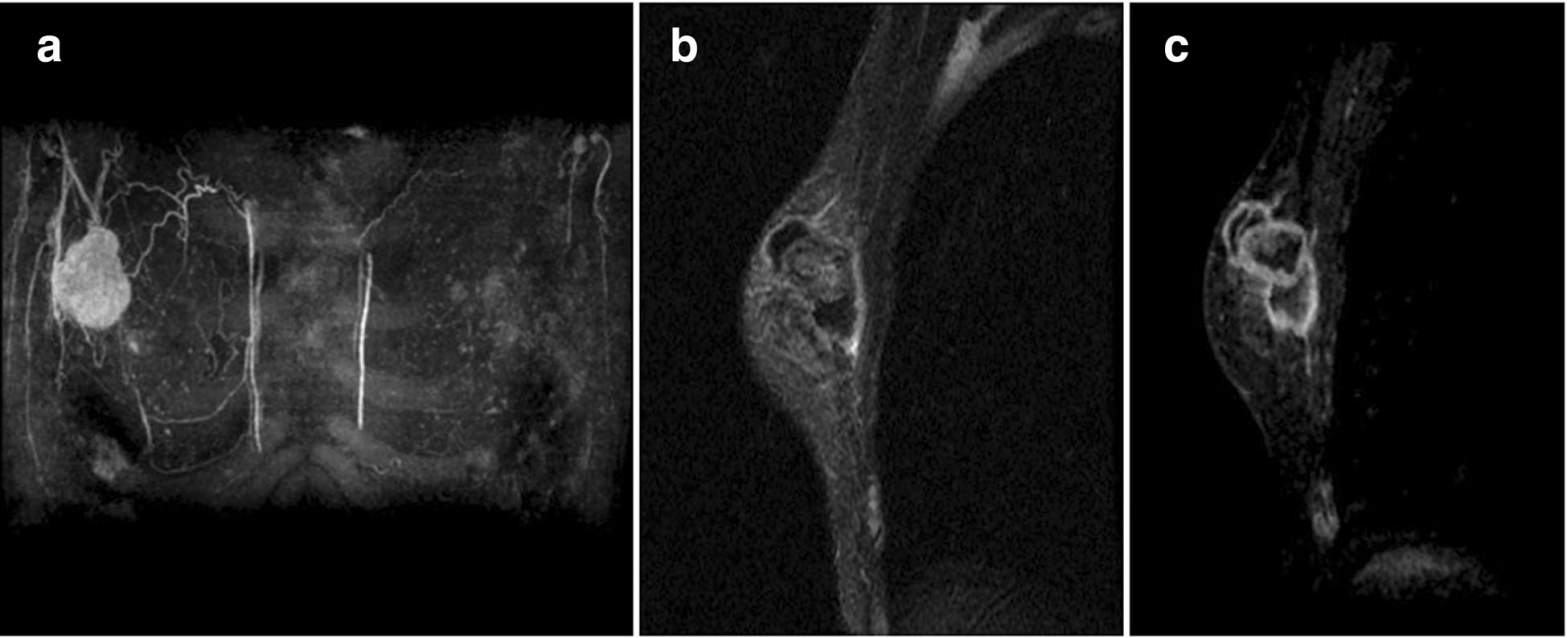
Magnetic resonance imaging. a A 4.5-cm-diameter mass at the upper outer portion of the right breast. b Fat suppression (FS) T2-weighted imaging (WI): A high signal intensity at the periphery and center of the tumor. c Gadolinium (Gd) FS T1WI: A high signal intensity at the periphery of the tumor but a low signal intensity in the central area
